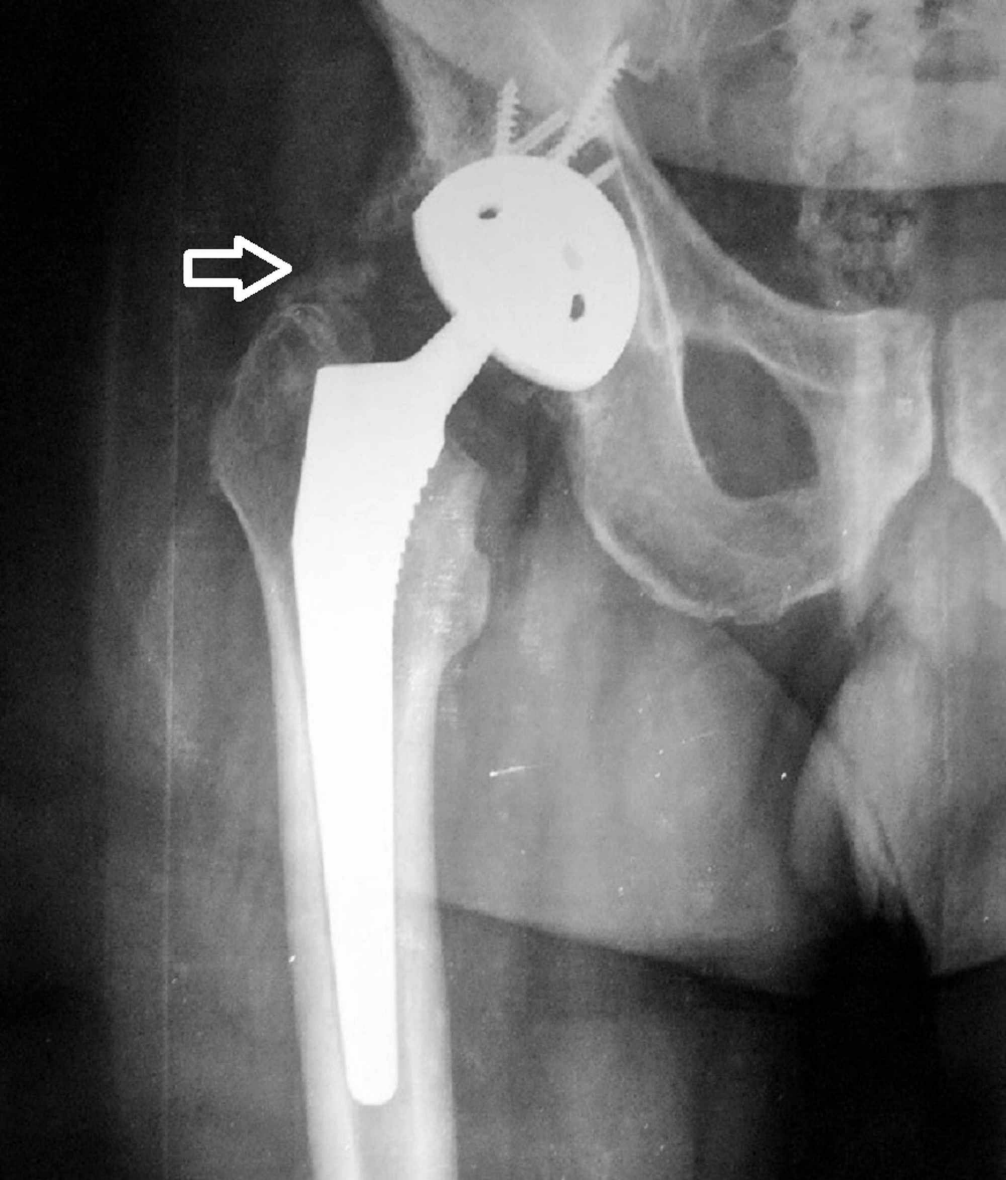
Radiograph of the same patient six years postoperatively (heterotopic ossification, Brooker III type, as marked by the white arrow)
